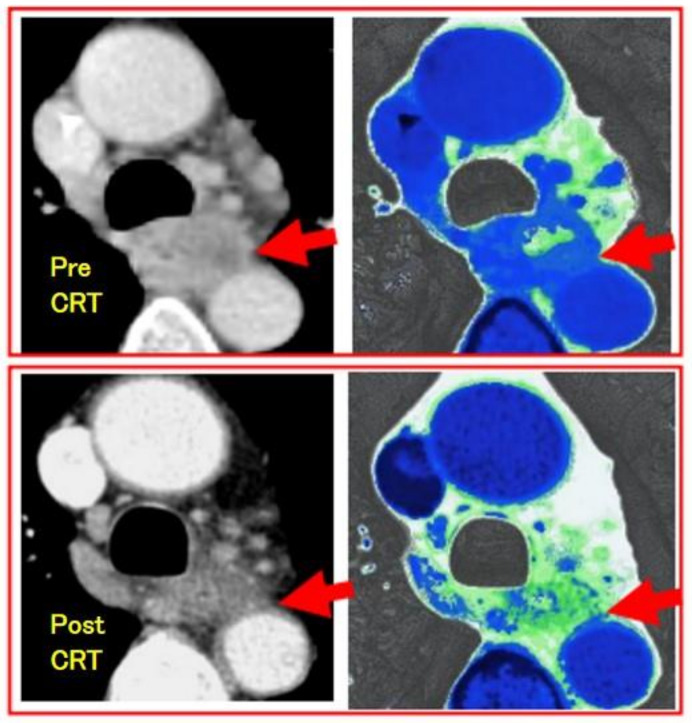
Downstaging evaluation using fibrous-tissue-enhanced CT reconstruction image of the case of esophageal squamous cell carcinoma after CRT. Contrast-enhanced CT was performed using multi-detector CT (GE light speed ultra). Arrows show the tumor. The upper is pre-CRT T4 image, which shows no interstitial plane between the tumor and aorta. The lower is post-CRT image, which shows fibrous plane as green and the case was diagnosed as downstaging and curatively resected with surgery.
